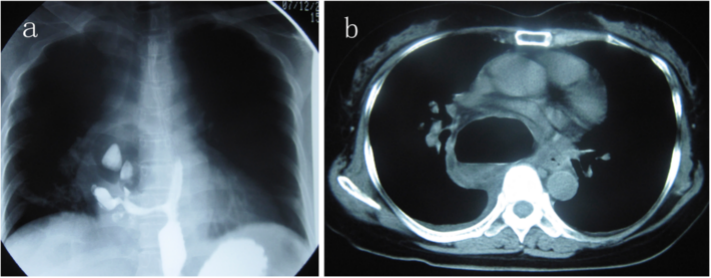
(a) A barium swallowrevealed a huge pare-esophagealcyst cavity compressed toesophagus,and also communicated toesophagus through a longentrance. (b) The chest CT scans showed a huge cystic mass full of gas and liquid located in the post-mediastinum with a direct compression of the pericardium, esophagus and right lung.
